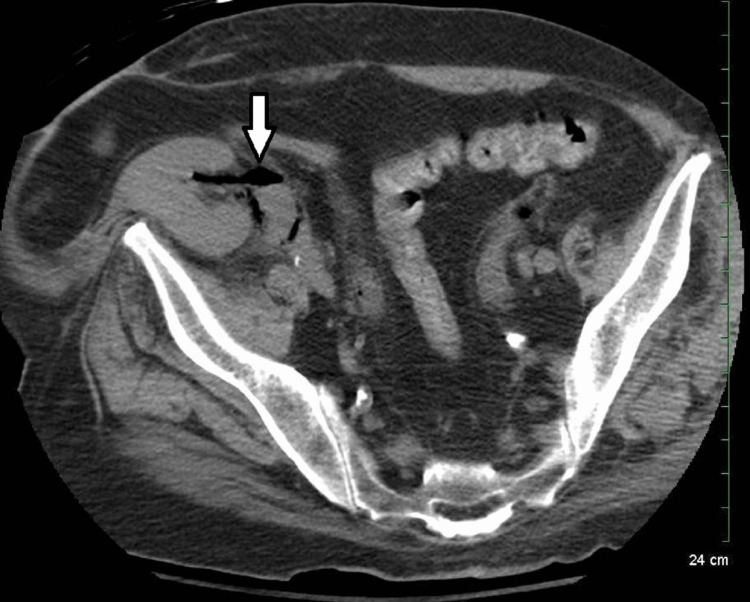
Non-enhanced CT image showing gas in the transplanted kidney proximal ureter (white arrow).
